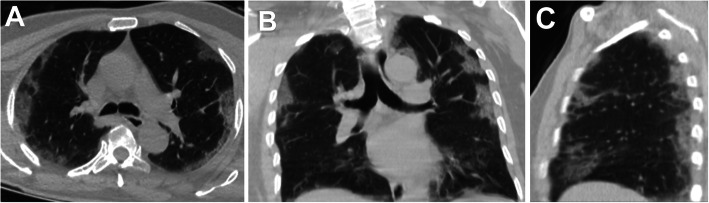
A 60-year-old female patient. A axial, B coronal, and C sagittal chest CT images show bilateral ground-glass opacities and consolidation with moderate pulmonary involvement (26–49%) corresponds to current chest CT score 13 and simple chest CT score 3 (moderate affection). The patient died on the seventeenth day
